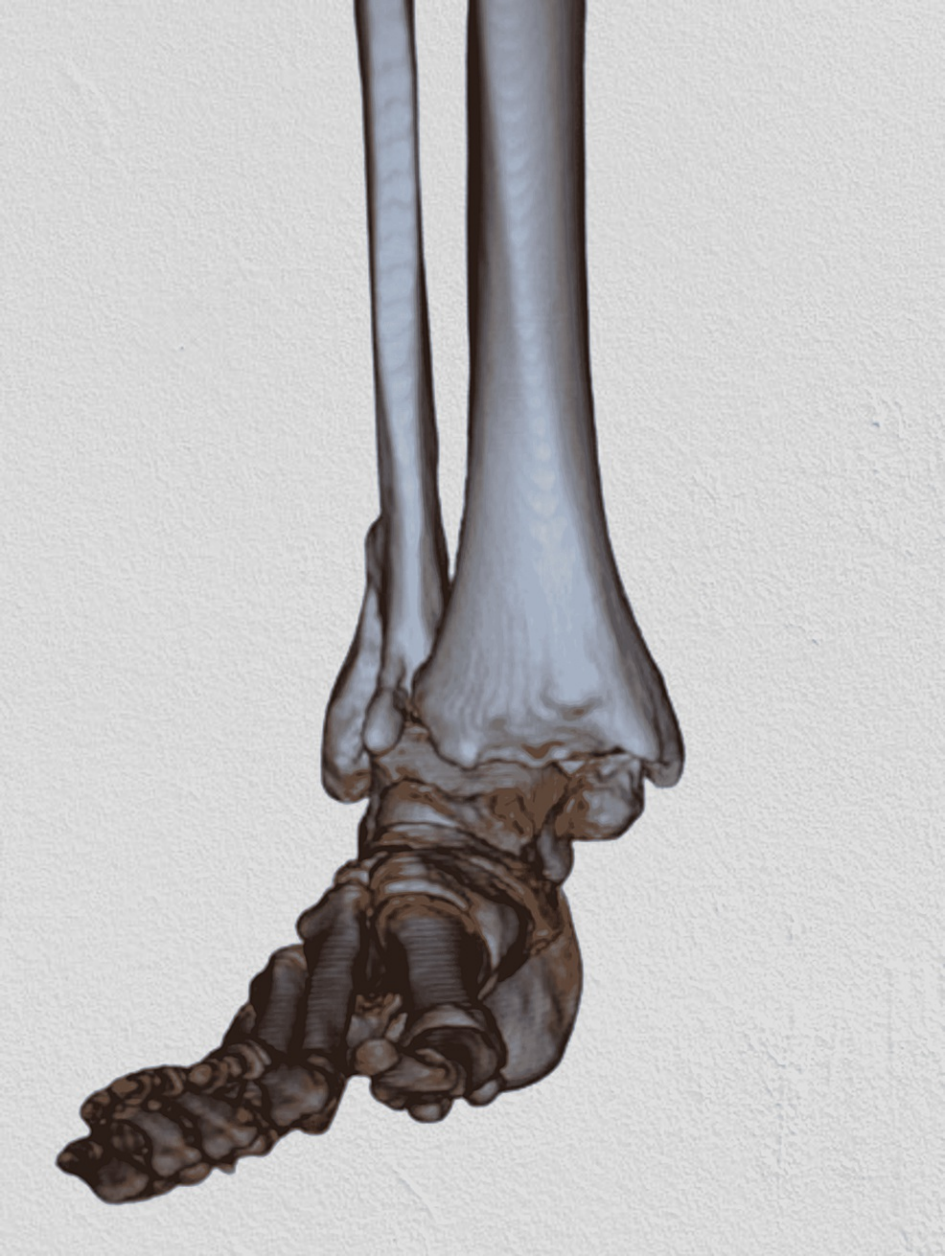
CT scan of the right ankle
